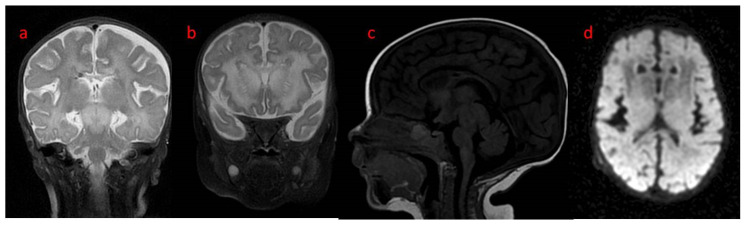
Brain MRI control after 1 week: (a,b) coronal T2: more generalized white matter T2 hyperintensity with a low signal rim around ventricles, marked symmetrical perivascular spaces in internal capsules; (c) sagittal T1 with an abnormal signal in cortico-spinal tracts in the brain stem; (d) axial DWI: slightly better signal differentiation with generally abnormal diffusivity.
